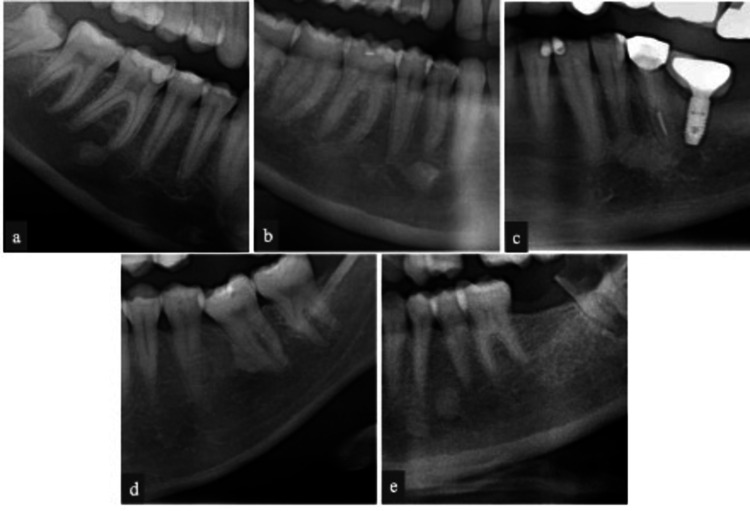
Panoramic radiographs showing osteosclerotic lesions related to teeth and root apices in different relations. (a) Apical, (b) separate, (c) apical interradicular, (d) interradicular, and (e) interradicular and separate.
